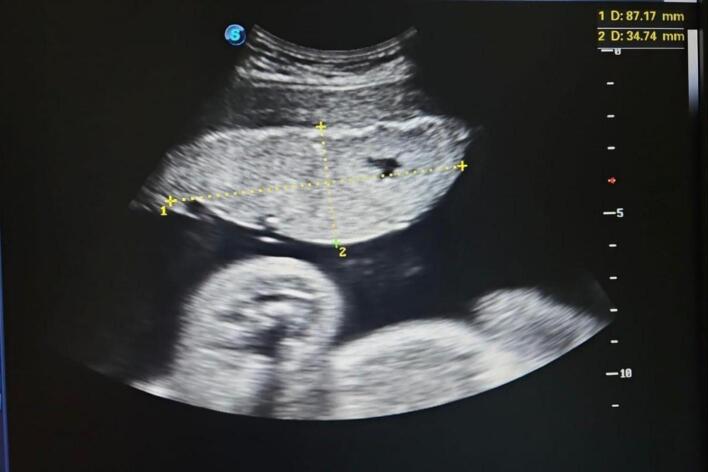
Ultrasound of bipartita placenta.
